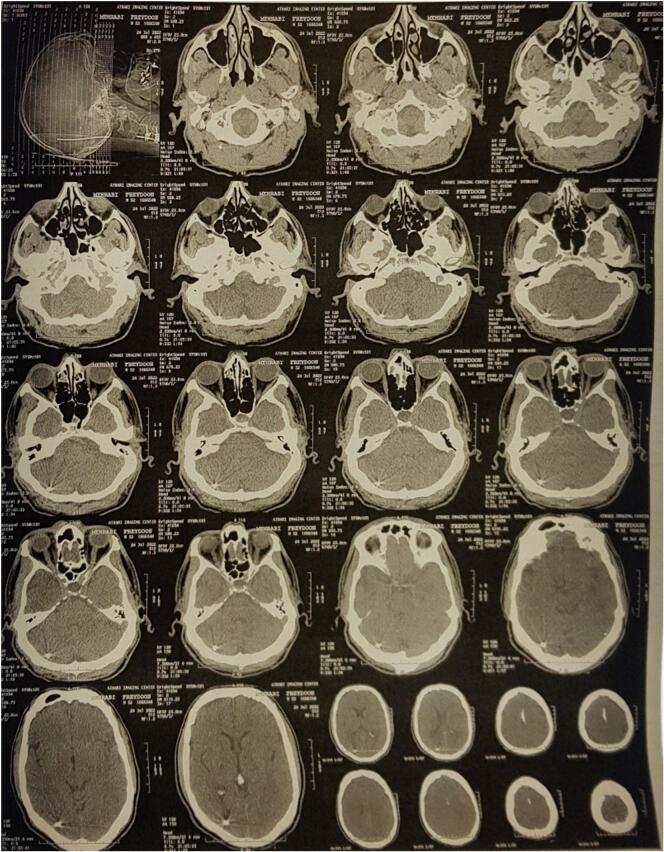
Axial CT scans of the brain, obtained four months postoperatively, showing the precise localization of the dislocated rod. The images highlight the migration path of the rod into the cranial cavity, with arrows indicating the rod's position relative to surrounding structures.
